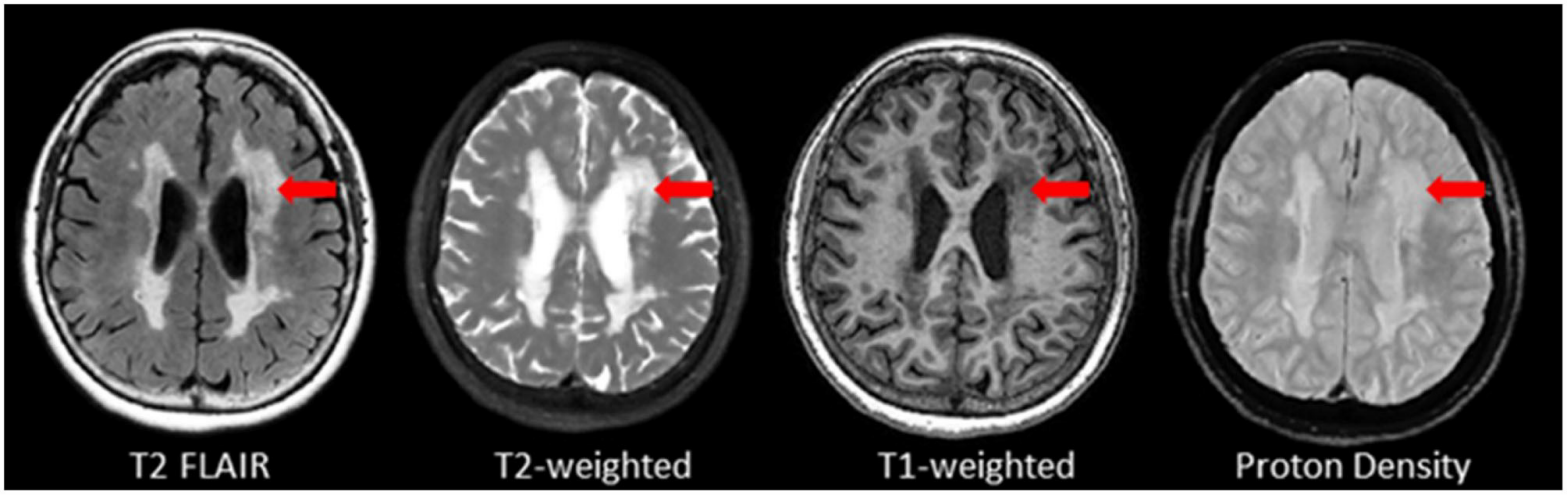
Axial view of various coregistered structural MRI sequences showing the relative intensity differences of WMH. Note that white matter hyperintensities are not hyperintense (i.e., bright) on T1-weighted MRI.
